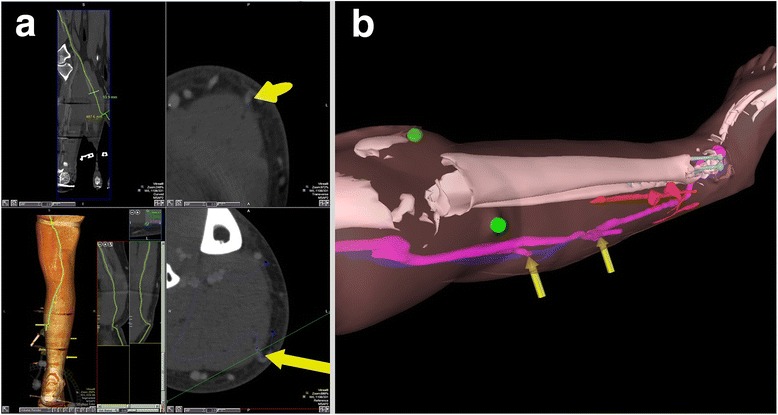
a Case 5 CTA imaging showing the location of perforating arteries with *yellow arrows*. b Case 2 example HoloLens rendering of segmented polygonal models
